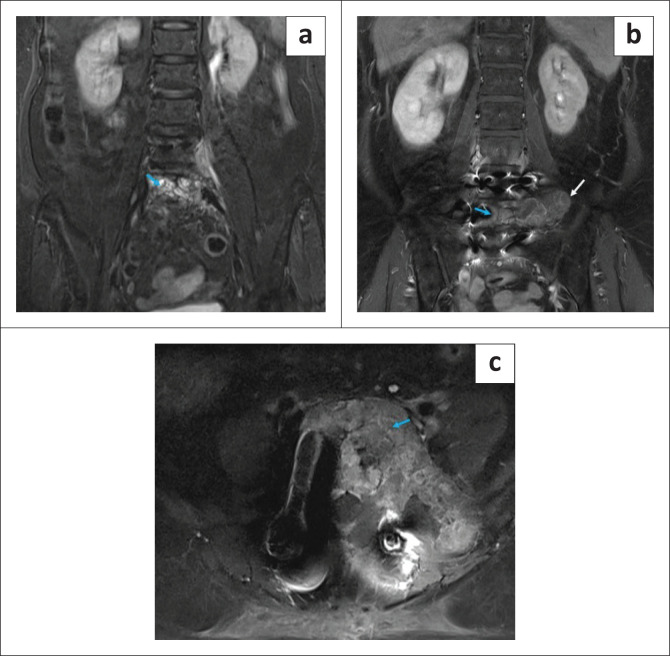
Post-operative MRI Short Tau Inversion Recovery (STIR) coronal, post-contrast T1 Fat Saturated sequence (T1FS) coronal and axial images. (a–c) A persistent expansile lesion is seen involving the body of the L5 vertebra (blue arrow). The lesion shows multiple hyperintense foci on the STIR sequence (a) and heterogeneous post-contrast enhancement on the T1FS sequence (b, c). (b) Residual soft tissue involvement is mainly on the left side (white arrow).
